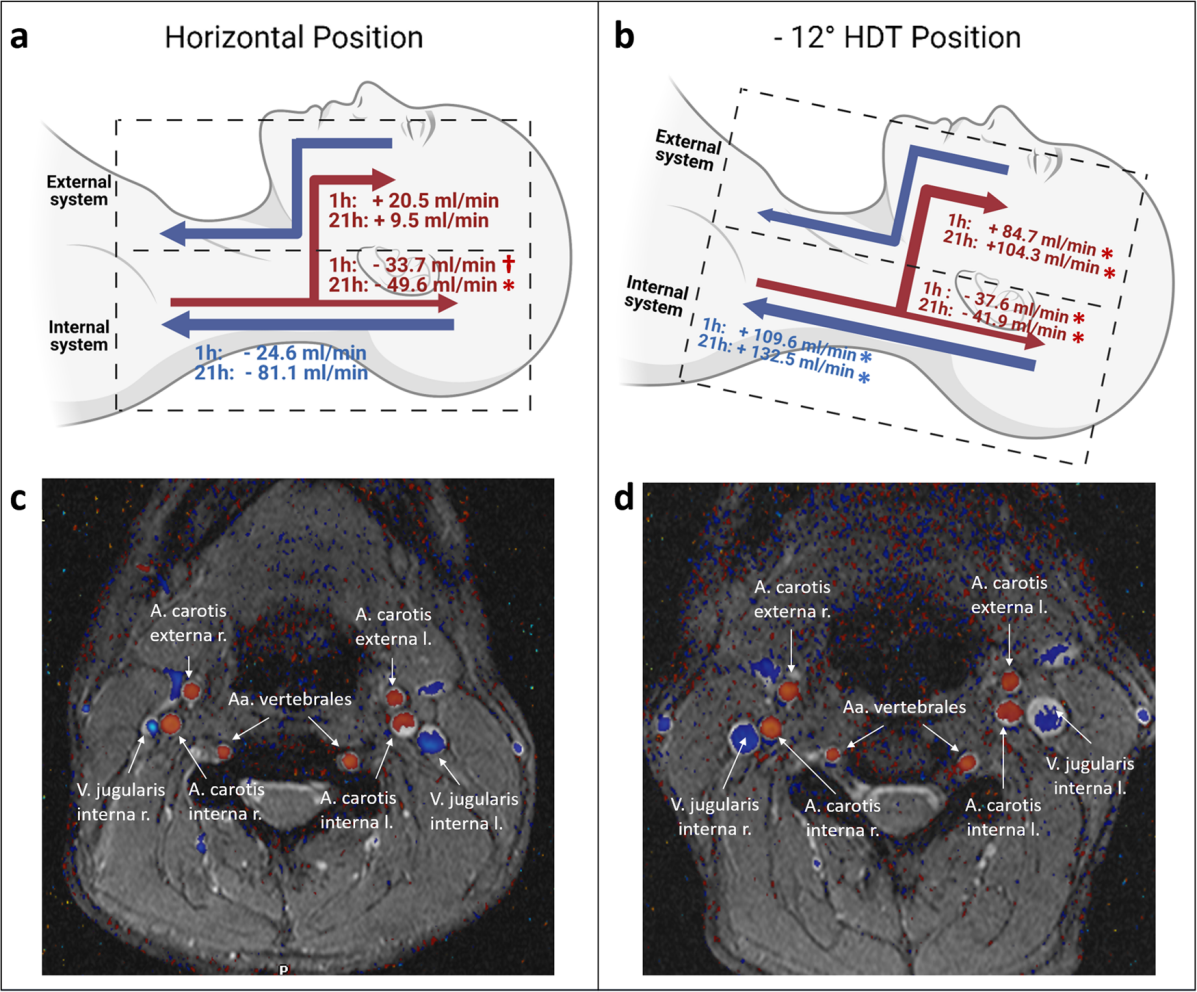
Difference in cerebral blood flow after 1 h and 21 h of the intervention. The numbers in panel a and b indicate the difference in flow in each respective position in comparison to the baseline data. The external system comprises the external carotid (red). The internal system includes the internal carotid artery (red) and jugular vein (blue). Algebraic signs refer to the physiological flow direction of each vessel. Figure panels c and d show anatomical MRI images of one of the subjects on the first interventional day in each of the respective body positions. †*P* < 0.05; P* < 0.001 (linear mixed effect models were used for statistical analysis). Panel a and b created with BioRender.com).
